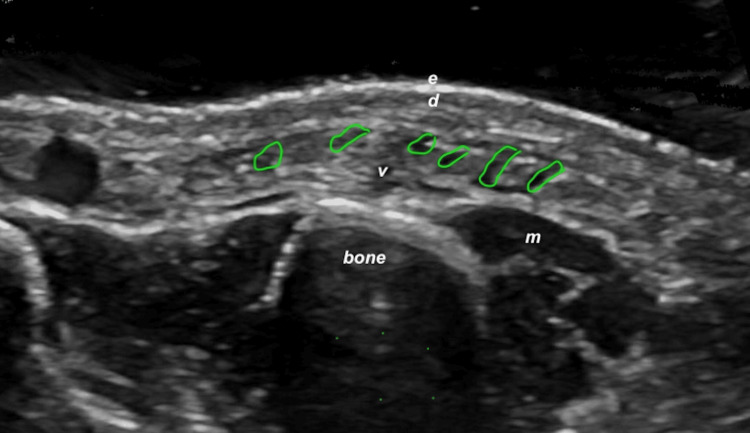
Sonographic appearance of HA filler in the dorsal hand (transverse view) Green boundary lines delineate multiple HA deposits with a characteristic pseudocystic, hypoechoic, laminar morphology. Epidermis (e), dermis (d), muscle (m), and bone (b) are labeled. Abbreviations: HA, hyaluronic acid
